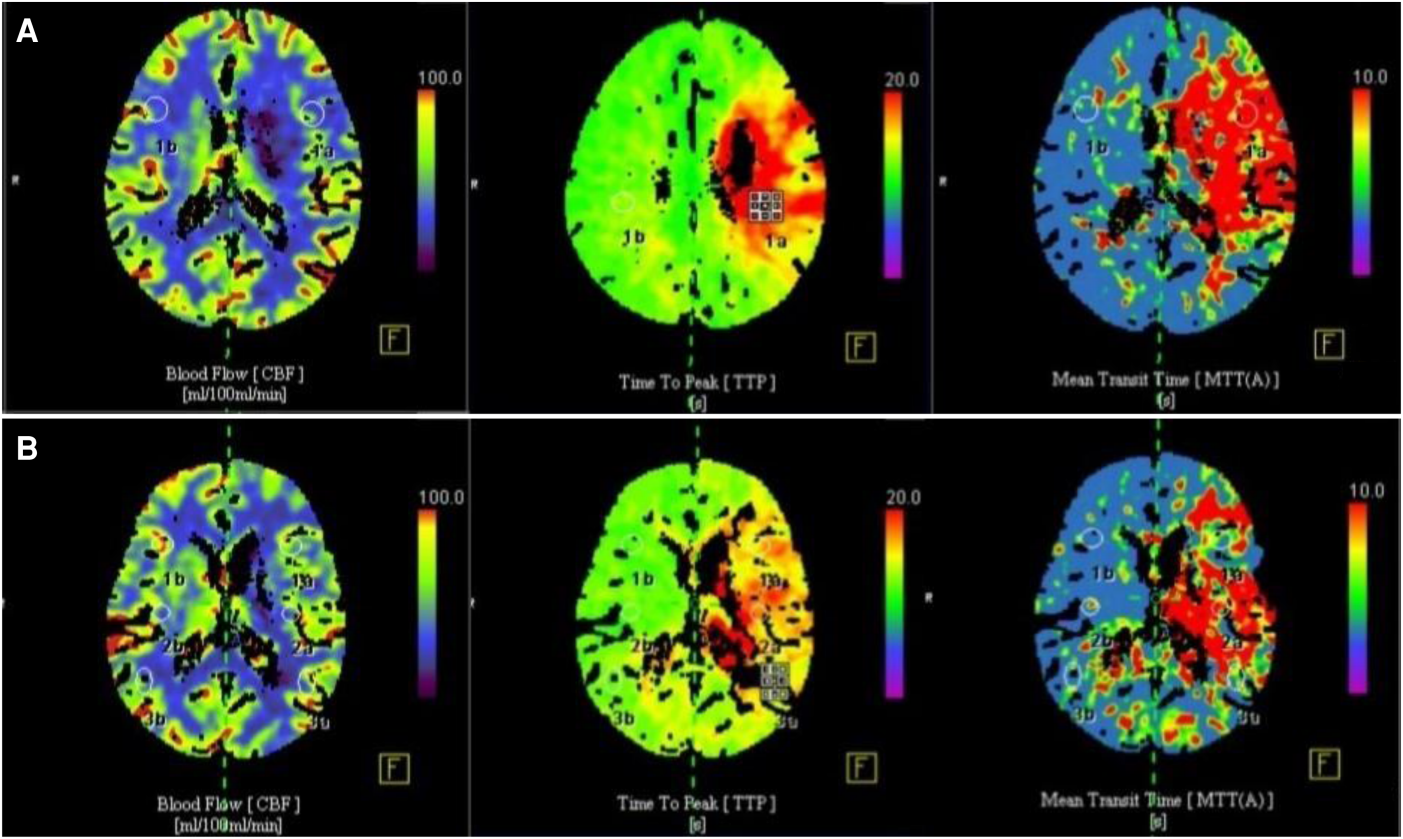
Comparison of CT cerebral perfusion parameters before and 3 months after surgery. (A) Preoperative cerebral blood flow was significantly reduced, CBF in cerebral perfusion CTP was significantly reduced, TTP and MTT were prolonged; (B) 3 months after operation, cerebral blood flow was significantly improved, CBF in cerebral perfusion CTP was increased, TTP and MTT time were significantly shortened.
